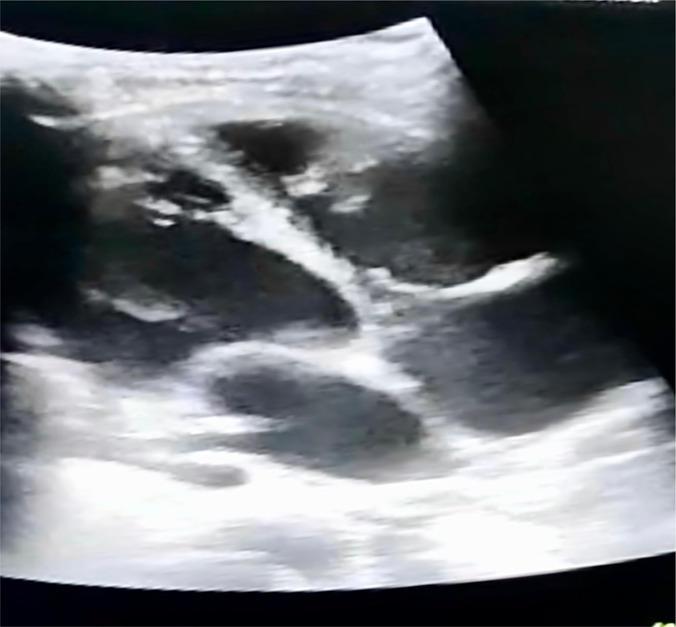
Bedside Echocardiogram: 4 chamber view showing BV dilatation on day 1 of admission
